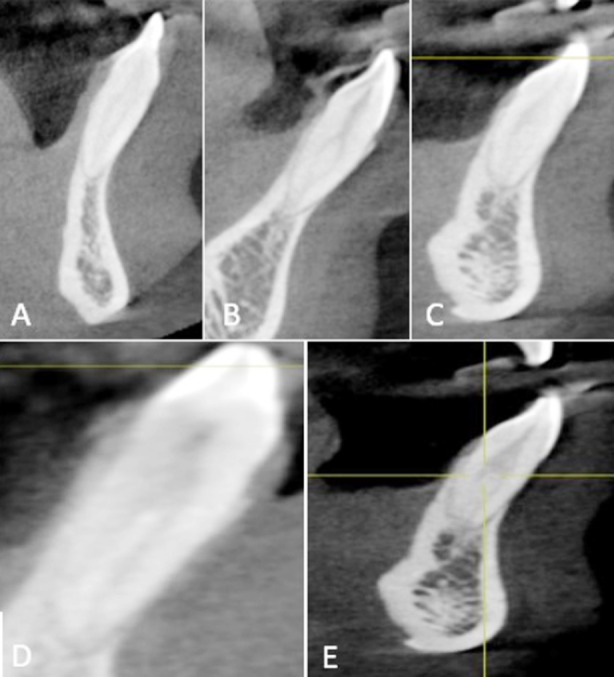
Mandibular incisors CBCT images with A- Type-II, B- Type-III, C- Type-IV, D- Type-V and E- Type-VII.
